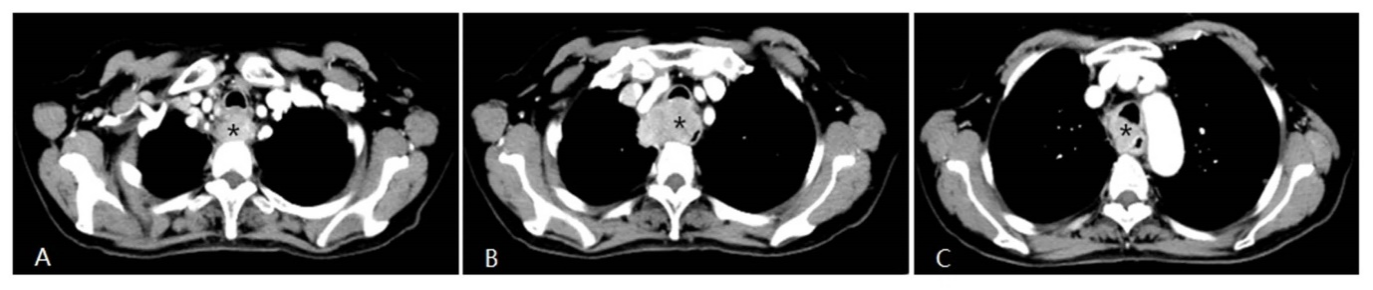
A patient with progressive dysphagia for 2 months was diagnosed with esophageal squamous cell cancer via endoscopic biopsy. Due to her poor condition, the oncologists did not recommend systemic chemotherapy, but instead suggested arterial infusion chemotherapy. Chest CT shows an apparently enhanced mass (*) in the middle-upper esophagus with moderate stenosis of the tracheal cavity due to tumor invasion.
